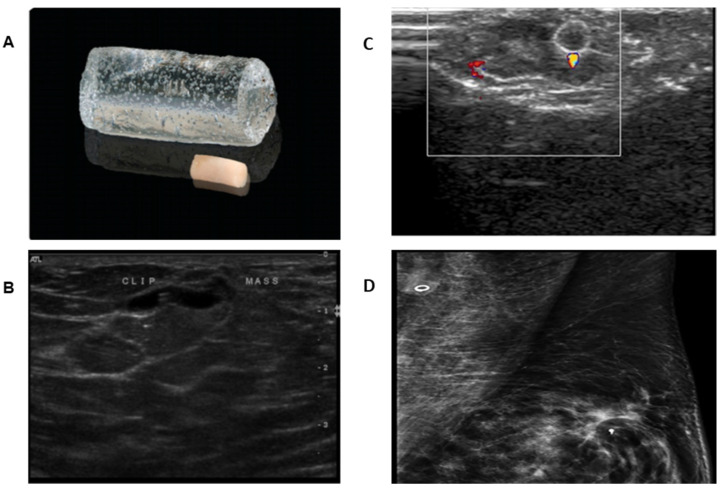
Sonographically visible HydroMARK clip. (A) Image showing cylinder shaped HydroMARK clip pre (small cylinder) and post (clear cylinder) absorption of water. (B) Transverse sonographic image of HydroMARK clip in the abnormal axillary lymph node. Typical sonographic view of HydroMark marker in the days following its implantation. From Woods et al. [33]. A review of options for localization of axillary lymph nodes in the treatment of invasive breast cancer. *Acad. Radiol*. 2019, *26*, 805–819 (Copyright obtained from the Rightslink Copyright Clearance Center under license number 4956080252494). (C) Sonographically visible Twirl marker, demonstrating clip with twinkle artifact within lymph node. (D) Presence of Twirl clip in left axillary node on mammogram. From Tan et al. [34]. The ‘twinkle’ artifact- a novel method of clip identification to facilitate targeted axillary surgery following neoadjuvant chemotherapy in breast cancer patients. *Clin. Imaging*
